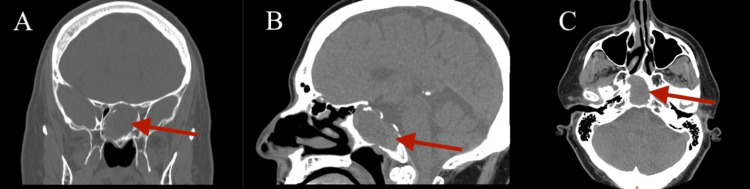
A computed tomography (CT) scan of the sinuses without contrast shows a large expansile mass measuring up to 3.5 cm centered on the sphenoid sinuses with extensive adjacent bony remodeling (red arrows). A. Coronal; B. Sagittal; C. Axial
